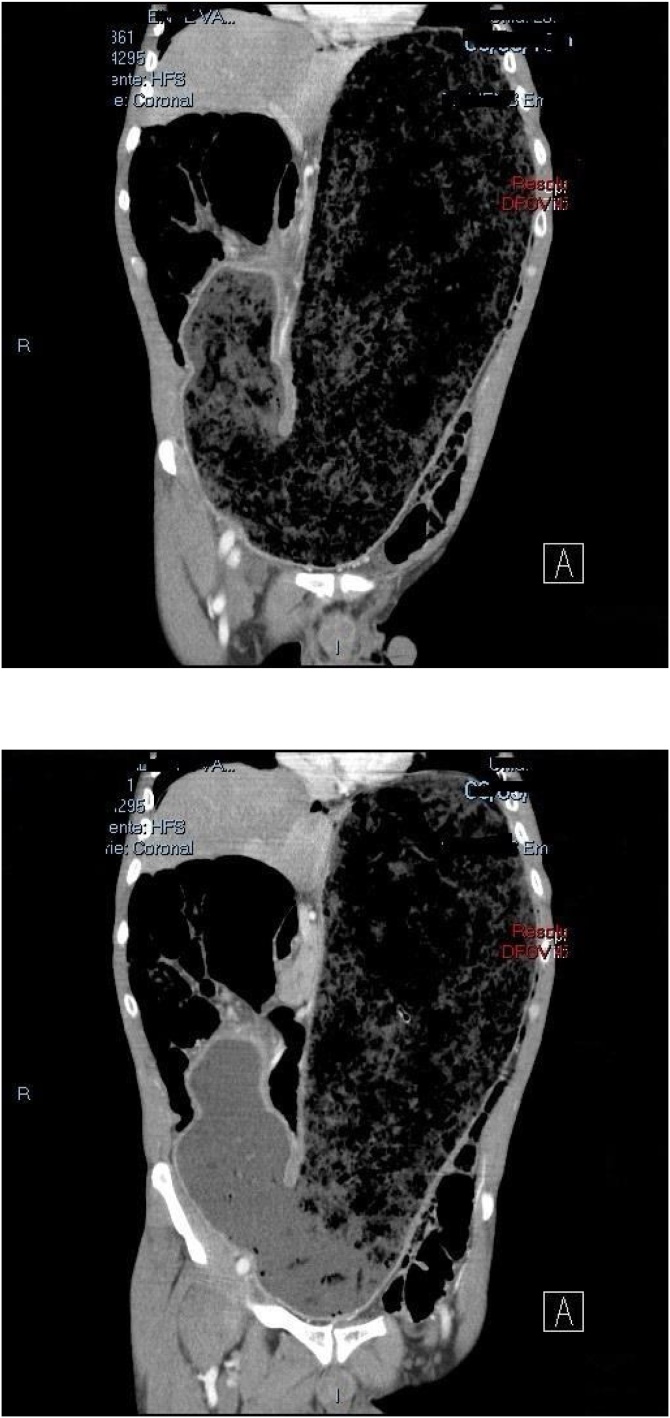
CT of massive gastric dilation due to pyloric stenosis (2018).
